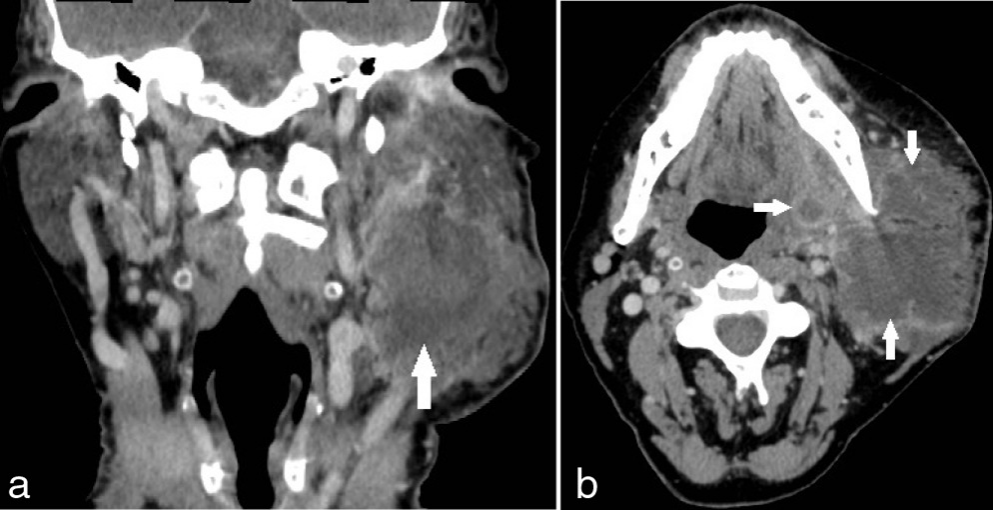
Preoperative venous phase contrast-enhanced CT images showing left side soft tissue enlargement of the face and neck with abscess cavities in (a) coronal reformat and (b) axial reformat.
